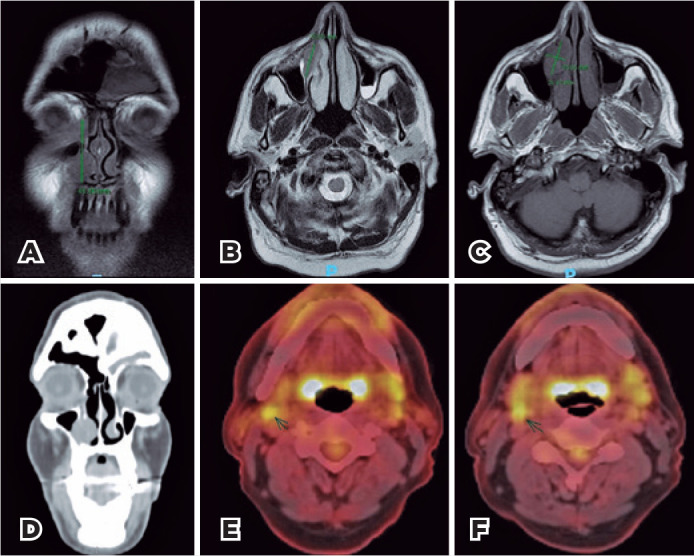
A) Coronal T1-weighted magnetic resonance imaging (MRI) demonstrates
